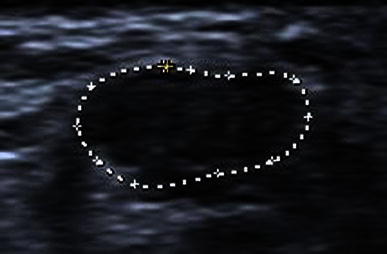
Anisotropy. Transverse image at the identical region of the same tendon as Fig. 2. Here the tendon appears far more hypoechoic. This can be misinterpreted as a defect but it is an ultrasound artefact due to the incident beam not being perpendicular to the tendon
